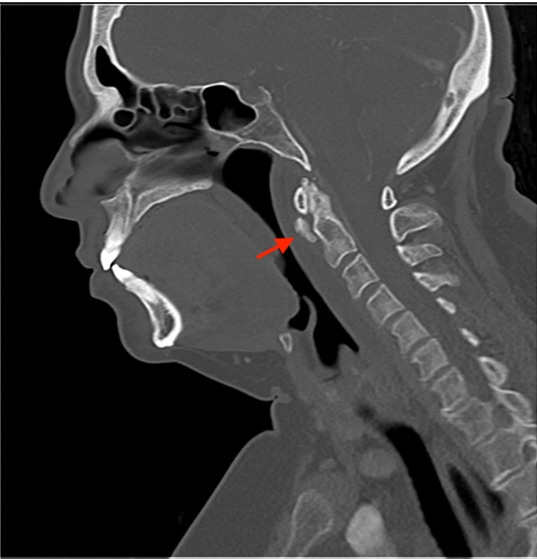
Sagittal computed tomography image in bone window showing focal calcification (arrow) in the longus colli tendon at the level of the first and second cervical vertebrae.
